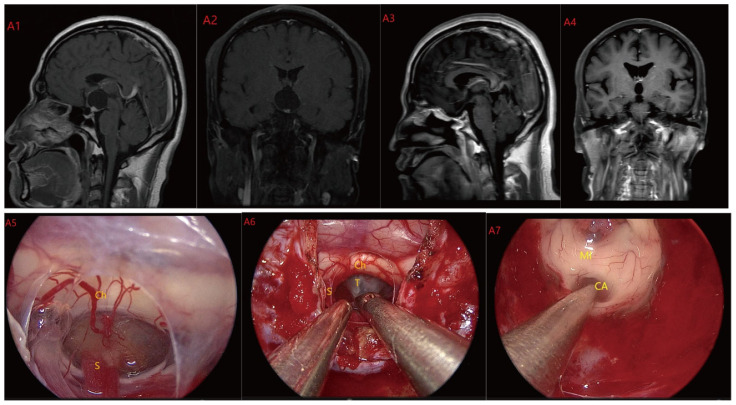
Case 2: Type II: The tumor located on the suprasellar region and compressed or penetrated the third ventricle floor (TVF) (A1,A2). During EEA surgery, the complete pituitary stalk can be seen via TCPCA, and the tumor is located behind and above the pituitary stalk (A5,A6). The tumor adhered closely to the floor of the third ventricle, and part of it protruded into the third ventricle during the operation. The tumor achieved GTR (A7). Postoperative MRI were shown in (A3,A4). Optic chiasm (Ch), foramen of Monroe, massa intermedia (MI), cerebral aqueduct (CA), tumor (T), pituitary stalk (S).
